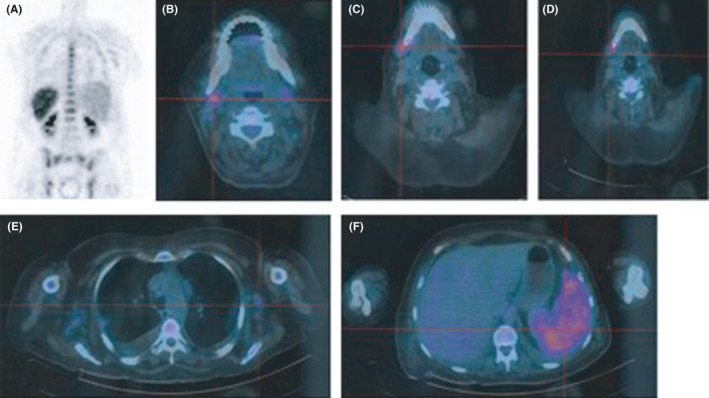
PET scan showing diffuse bone marrow (A), lymphadenopathies—retromandibular (B), submandibular (C), cervical (D), axillar (E), and spleen (F) increased uptake. (SUVmax: 5.64 at right cervical adenopathies)
